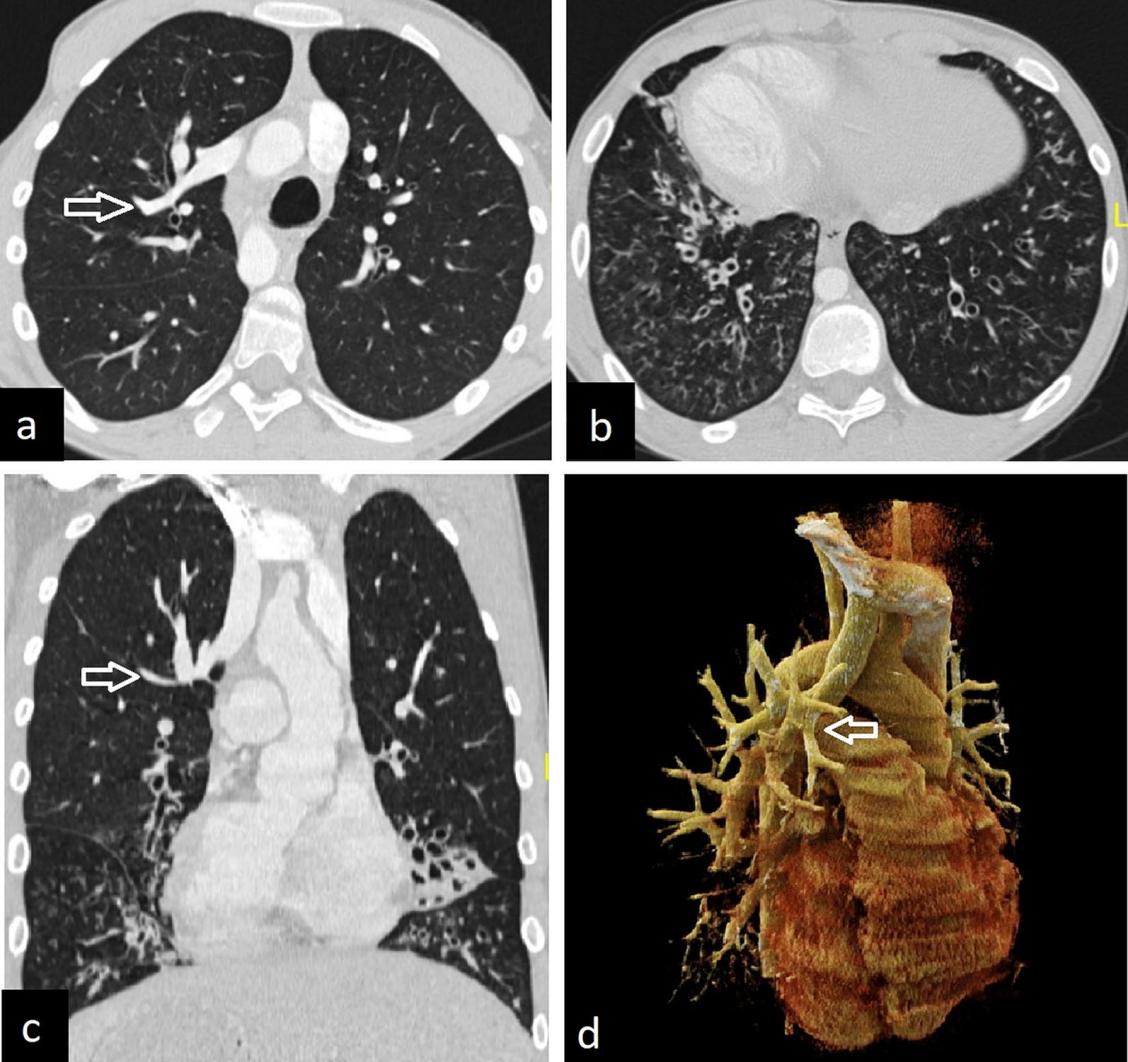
Mixed lesions-Kartagener syndrome and partial anomalous pulmonary venous return (PAPVR) in a 20-year-old man. Axial (a) and coronal (c) CT images show anomalous right upper lobe pulmonary veins draining into the right brachiocephalic vein. Bronchiectasis, bronchial wall thickening, and centrilobular opacities predominantly affecting lower lobes of the lungs are seen on axial CT image (b). Coronal CT image (c) demonstrates both PAPVR (arrow) and bronchiectasis. 3D volume-rendered reconstruction image (d) shows PAPVR (arrow)
